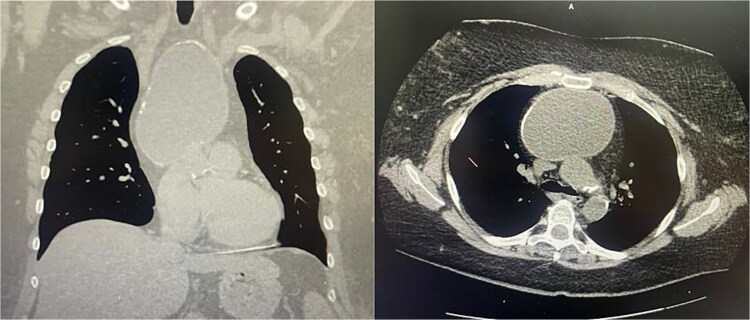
Coronal and axial views of a non-contrast CT of the chest demonstrating a large fluid density in the anterior mediastinum with rim calcification, subtle septations, and some nodularity, measuring 7.1 × 9.2 × 11.2 cm, accompanied by multiple small bilateral pulmonary nodules.
